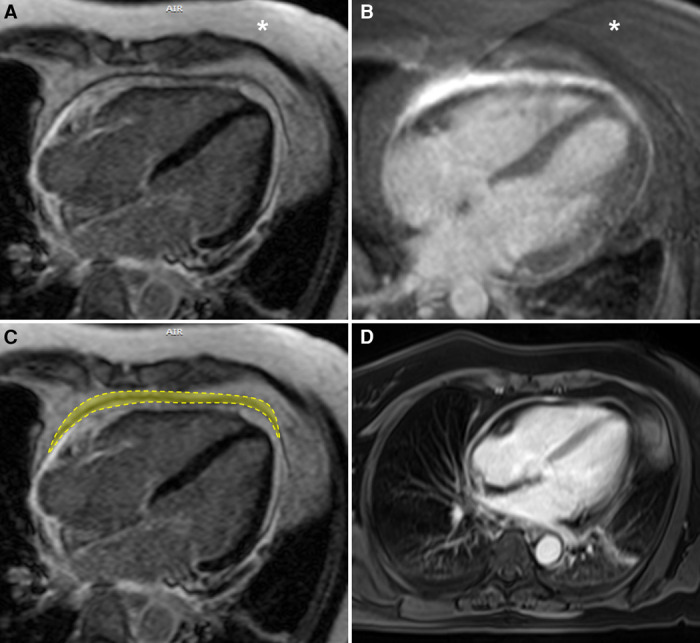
Forty-year-old male presented with acute chest pain. Initial evaluation excluded coronary artery disease. MRI was requested to evaluate for myocarditis and showed pericardial thickening and enhancement. A significant difference can be seen between the areas of hyperintense signal in LGE sequences without and with fat saturation (*A* and *B*, respectively). Note the difference in subcutaneous fat signal intensity (asterisk) in *A* and *B* to differentiate between the non-fat-suppressed and fat-suppressed sequences, respectively. The area of ‘true’ pericardial enhancement (outlined) appears markedly reduced in the sequence without fat saturation (*C*). Pericardial thickening and enhancement can also be characterized in post-contrast non-gated Dixon-type T1-weighted sequence (*D*). Technical parameters: (*A*) non-fat-saturated inversion recovery prepared gradient echo pulse sequence (TR = 881.6 ms, TE = 4.72 ms, FOV = 380, matrix = 232 × 256, flip angle = 25°); (*B*) fat-saturated inversion recovery prepared gradient echo pulse sequence (TR = 630.6 ms, TE = 1.74 ms, FOV = 400, matrix = 232 × 256, flip angle = 20°); and (*D*) dual-echo volumetric interpolated breath-hold examination (VIBE)-Dixon T1-weighted sequence (TR = 6.8 ms, TE1 = 2.39 ms, TE2 = 4.77 ms, FOV = 380, matrix = 256 × 320, flip angle = 8°).
